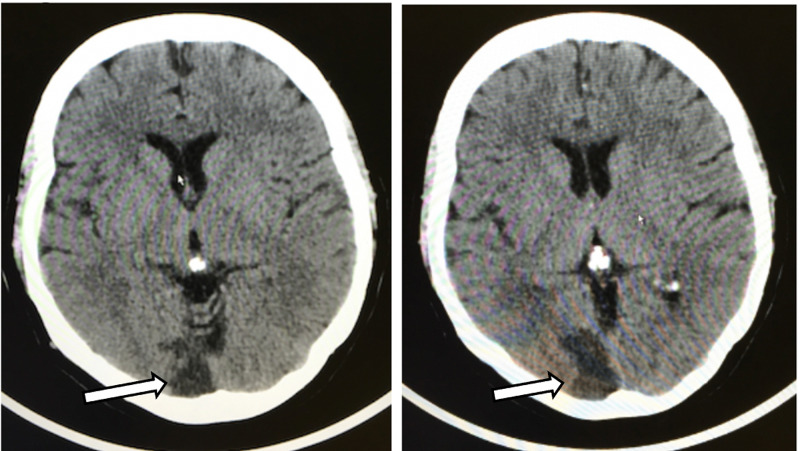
Computer Tomography of Brain Computed tomography of brain without contrast demonstrating right posterior infarction (white arrows).
